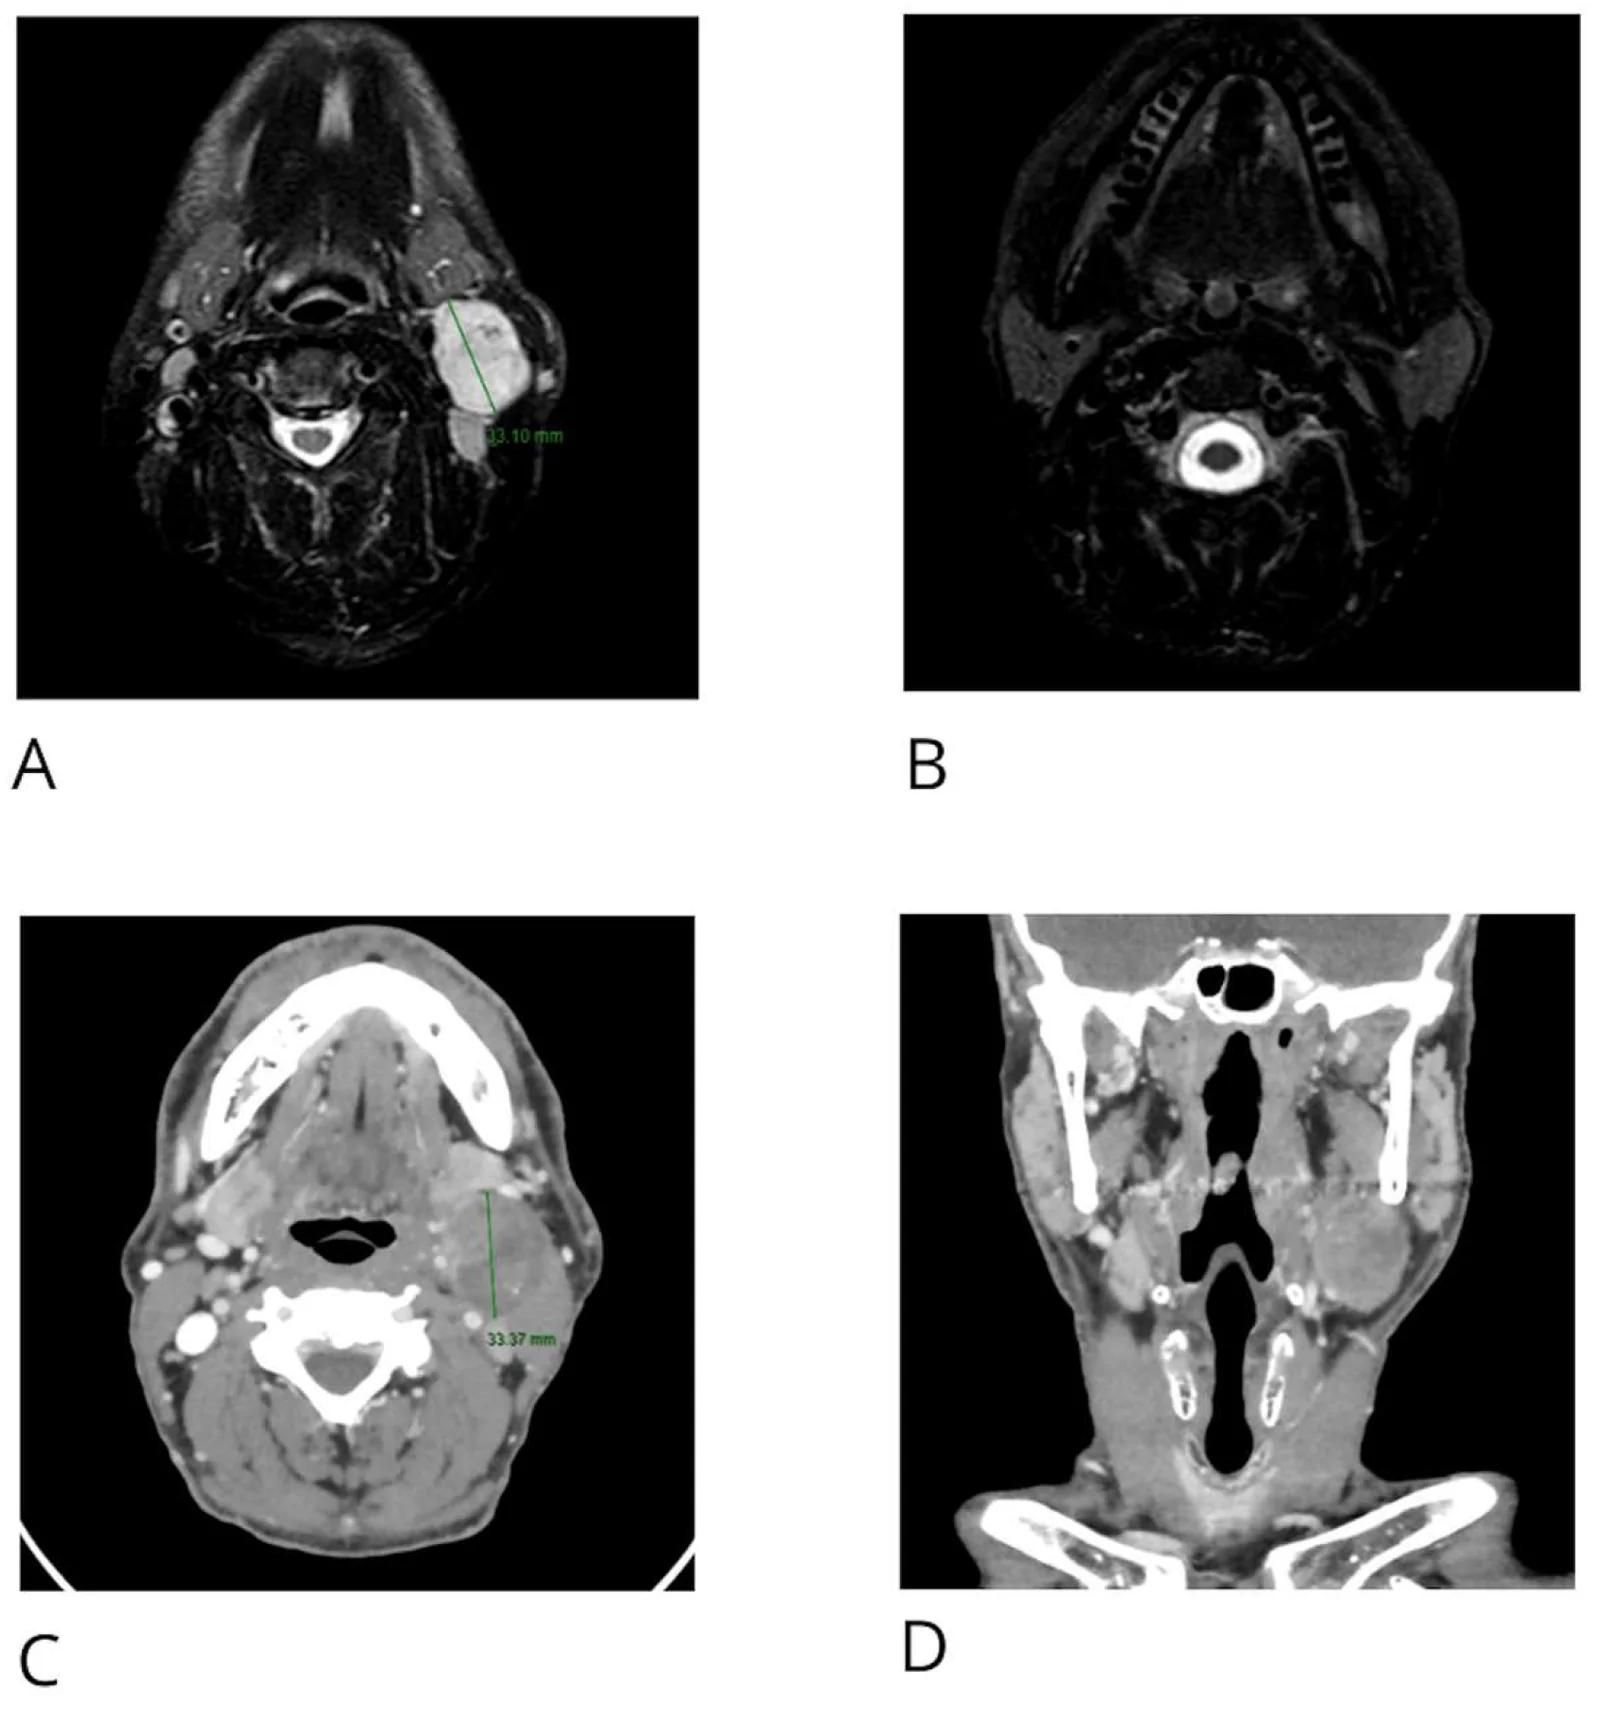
Preoperative imaging. (A) Axial T2-weighted MRI showing a suspicious left level IIA lymphadenopathy with a maximum diameter of 33 mm. (B) Axial T2-weighted MRI demonstrating a poorly defined area of altered signal at the left palatine tonsil. (C) Axial CT, confirming the presence of a 33 mm laterocervical mass. (D) Coronal CT highlighting the laterocervical lesion.
